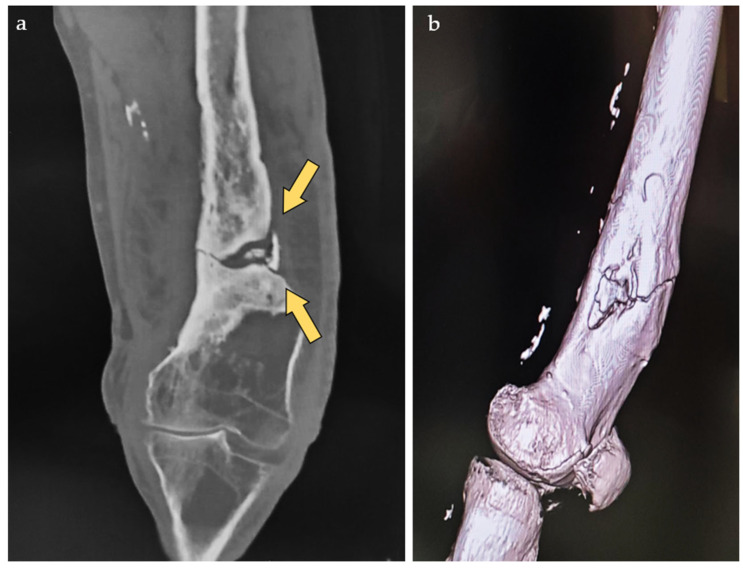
Preoperative CT scan with reformatting in the coronal plane (a) and virtual rendering technique (b) depicting the non-united fracture (yellow arrows).
